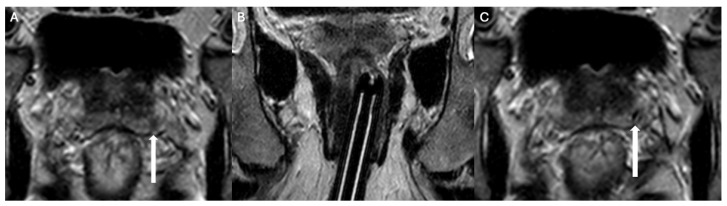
Direct MRI-Guided Targeted Biopsy Procedure of a PI-RADS 4 Index Lesion. (A) Axial T2-weighted MRI: index lesion in the mid-glandular left peripheral zone (arrow). (B) Coronal T2-weighted sequence: transrectal probe aligned toward the index lesion. (C) Intraprocedural axial T2-weighted image: focal gadolinium hypointensity at the needle tip confirms correct intralesional placement (arrow).
